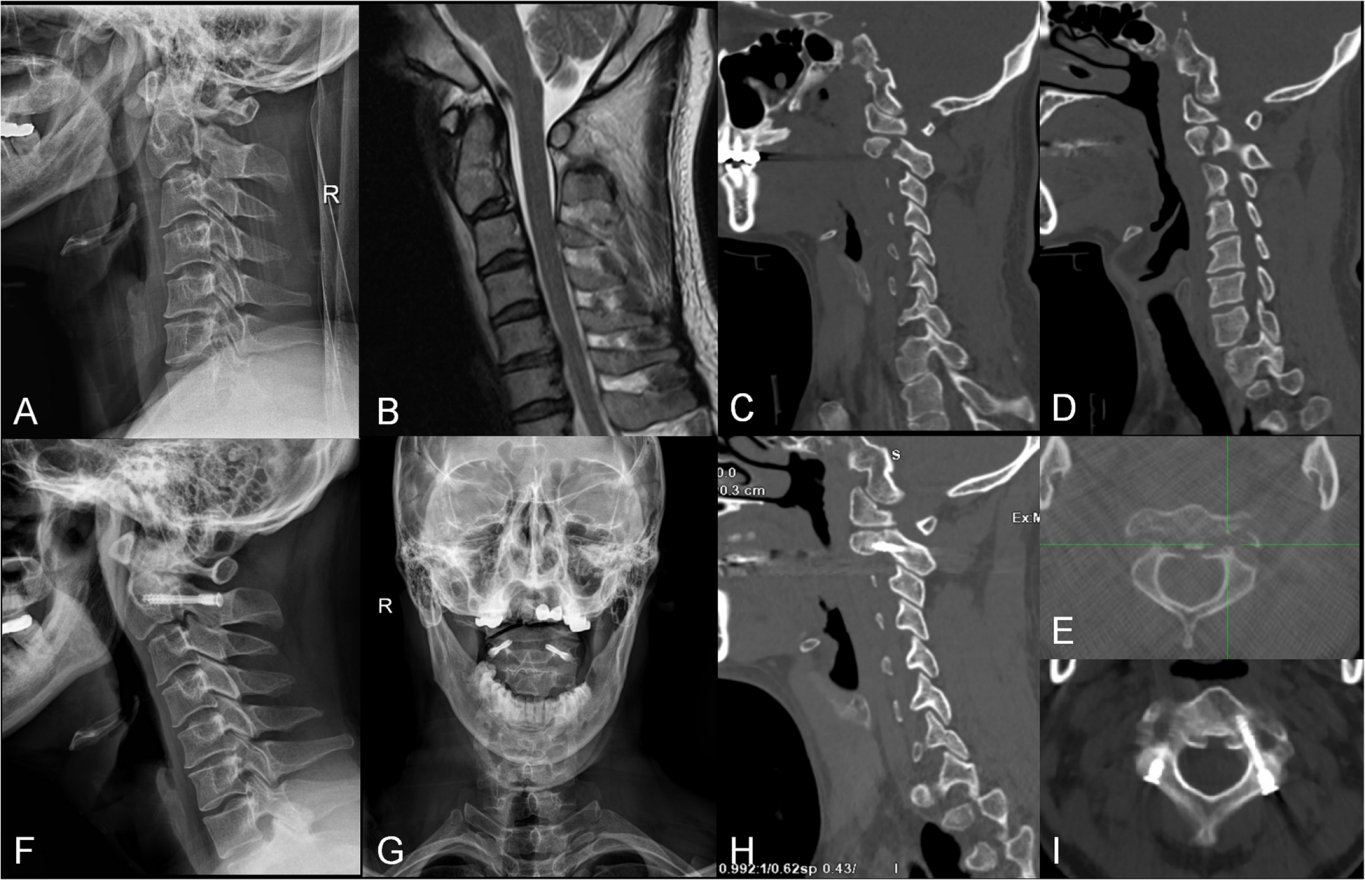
a The preoperative lateral radiograph of a 32-year-old male patient with a type IIA Hangman’s fracture. b A preoperative sagittal T2-weighted magnetic resonance image (MRI) revealed the absence of spinal cord compression. c–e The preoperative computed tomography (CT) scan reconstructions showed an obvious separation of fracture on both sides. f, g The postoperative lateral and open-mouth radiographs showed adequate fracture reduction. h, i The CT scan reconstructions at the 3-month follow-up showed satisfied osseous union
